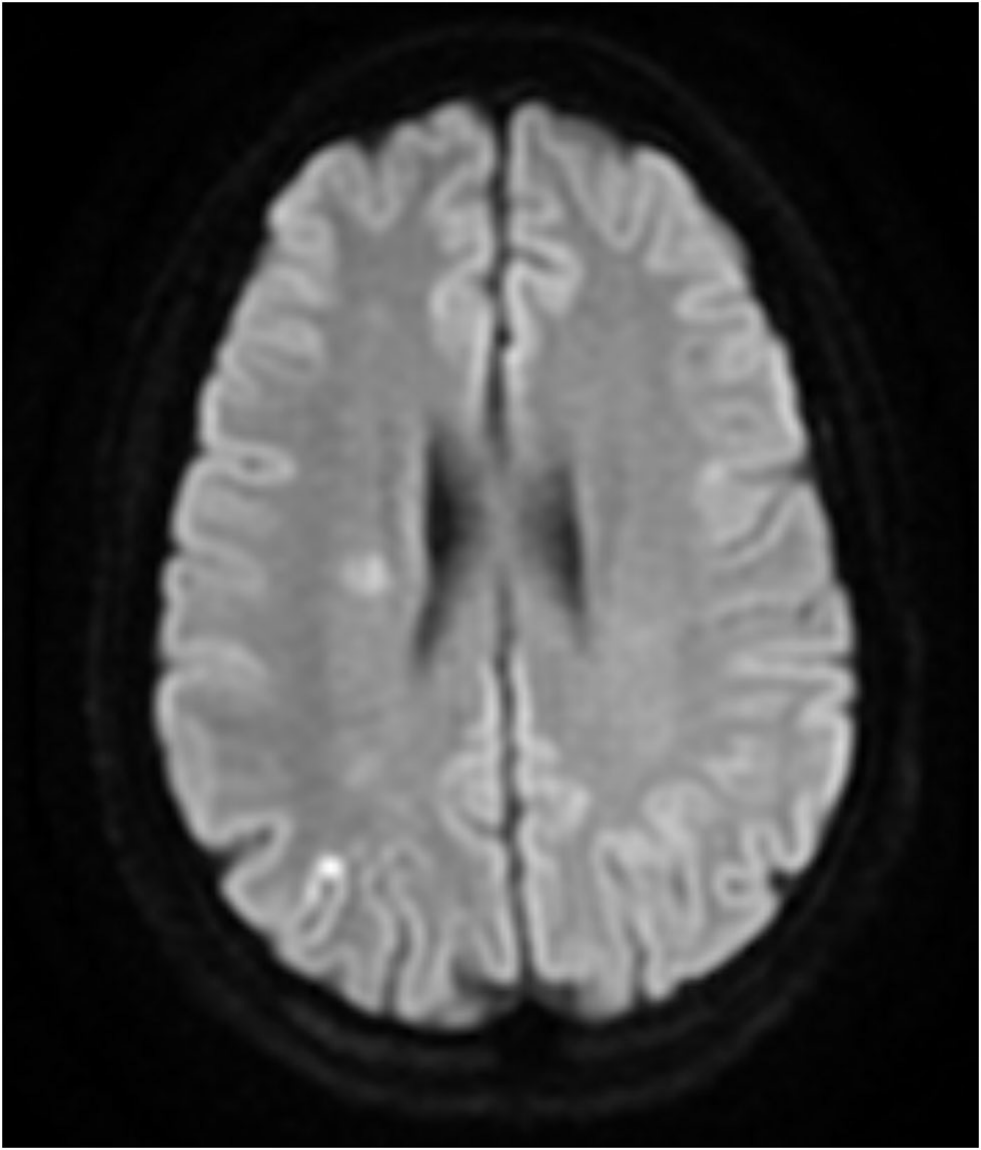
MRI DWI sequence of Mixed Infarct.
